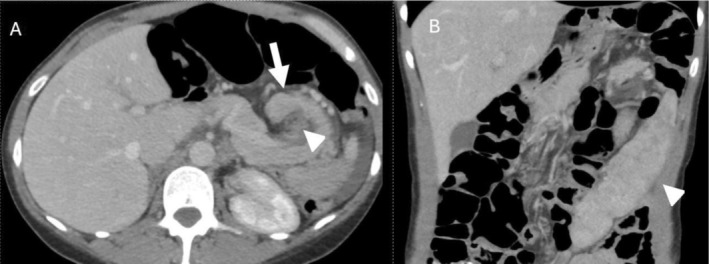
(A) Axial and (B) coronal contrast enhanced CT images. (A) Partial detorsion of pancreatic tail (arrow) with reduced swirling at the splenic hilum and improved enhancement. Fatty infiltration related to pancreatitis is improved (arrowhead). (B) The spleen (arrowhead) remains enlarged but shows partial detorsion and decreased swirling, pelvic position and improved enhancement.
